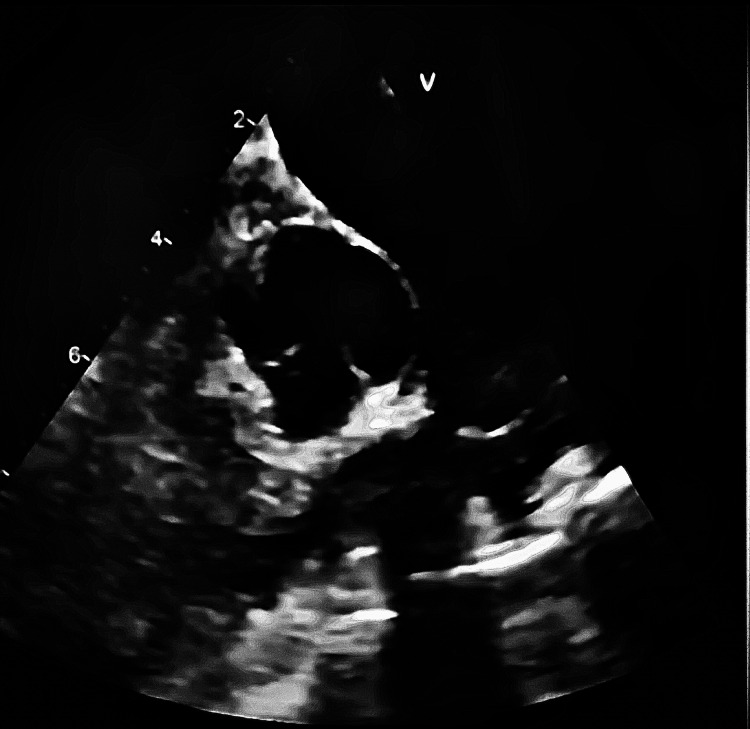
Intraoperative echocardiographic view of the pulmonary valve during surgical intervention. Intraoperative transesophageal echocardiographic image showing the prosthetic pulmonary valve region and right ventricular outflow tract. The image was optimized for resolution and labeling; however, quality reflects the inherent limitations of intraoperative acquisition.
